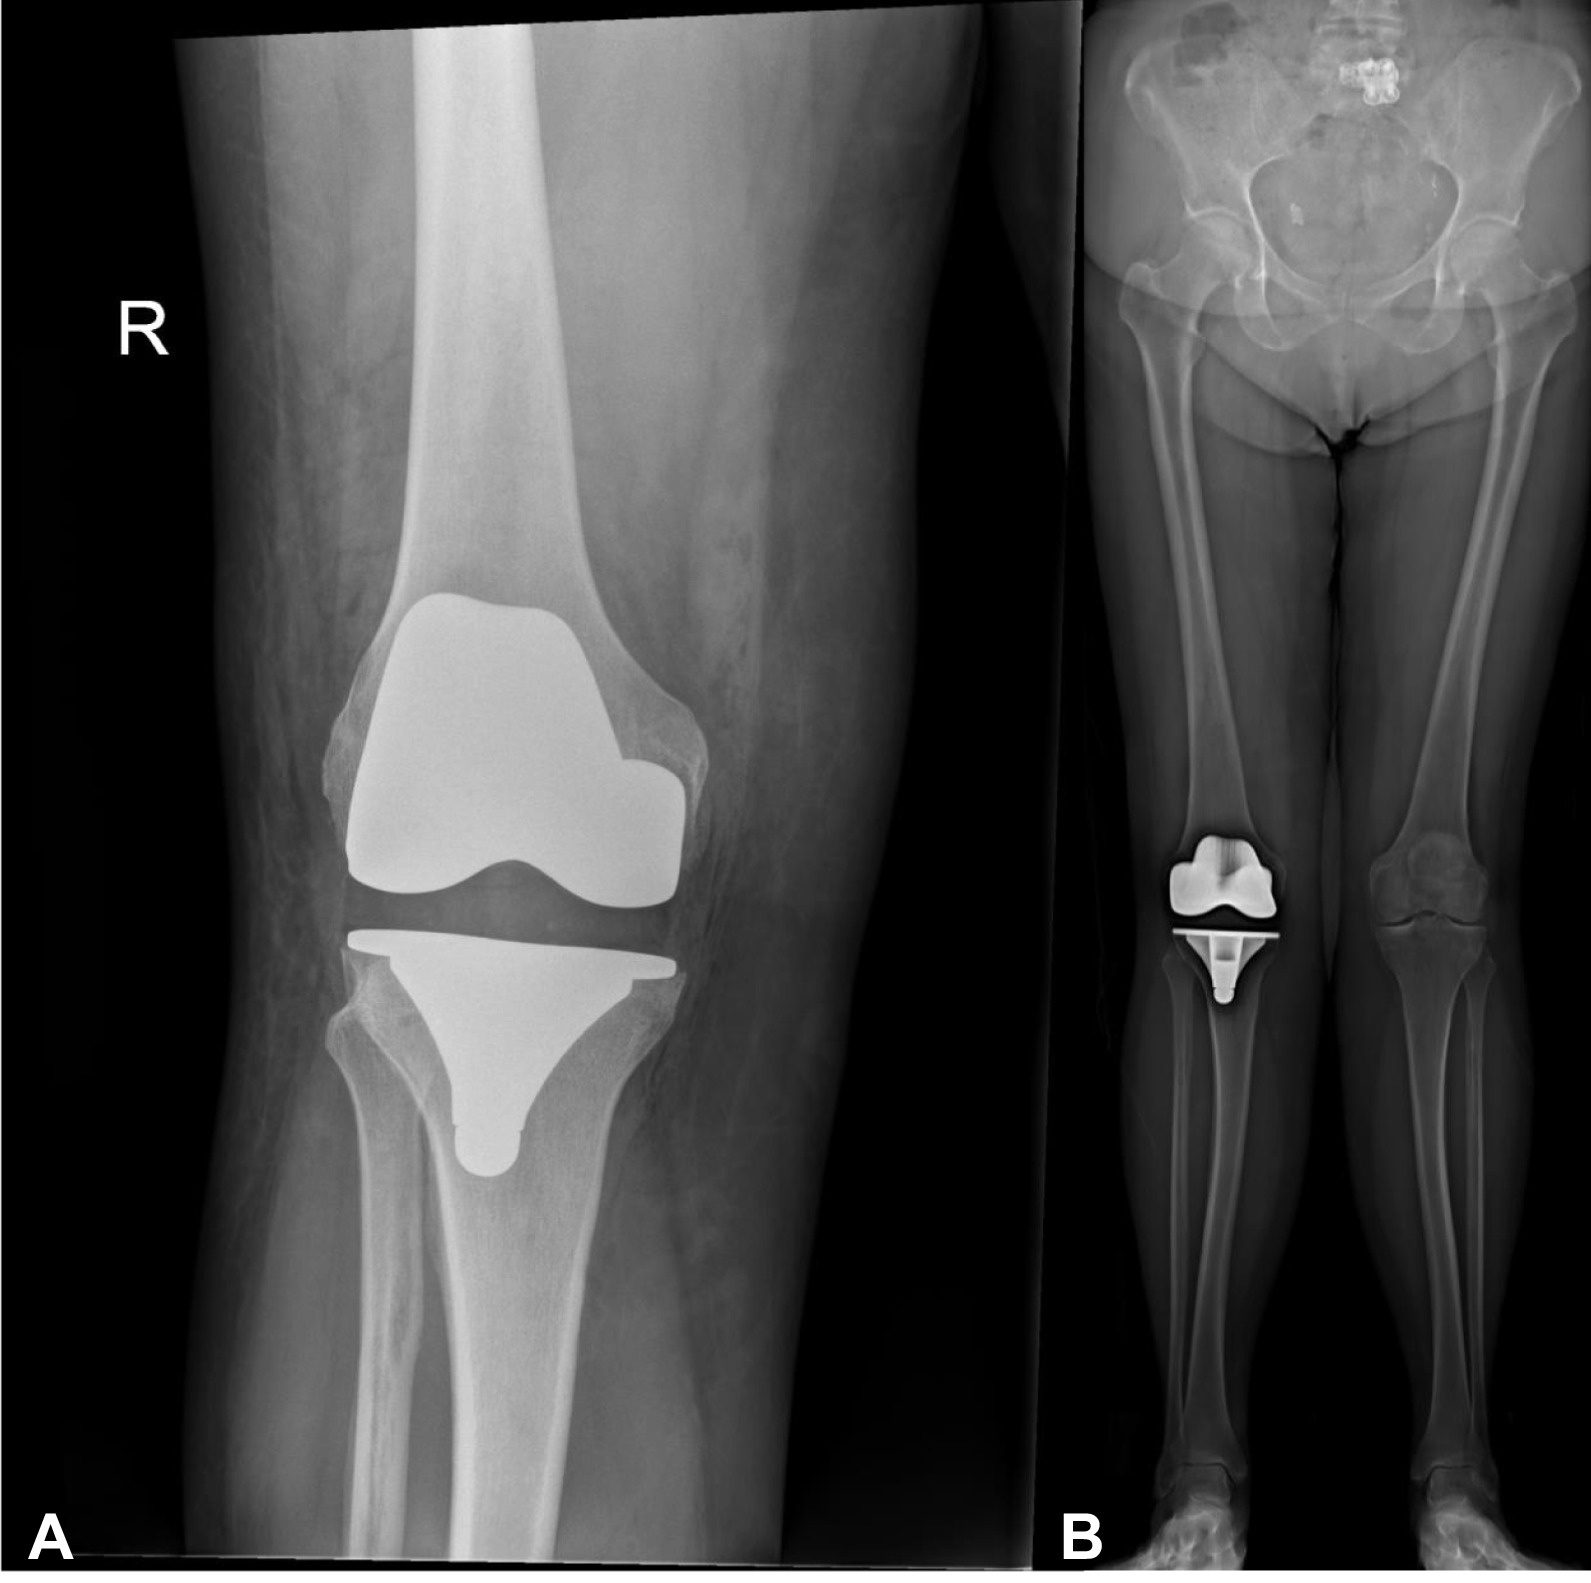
Clinical significance of full lower-limb weightbearing radiographs in total knee arthroplasty (TKA). A Post-TKA anteroposterior radiograph of the right knee demonstrating satisfactory position. B Same patient with a post-TKA weightbearing lower-limb anteroposterior EOS digital radiograph, demonstrating an extra-articular tibia valga deformity and post-operative valgus alignment of the right lower limb.
